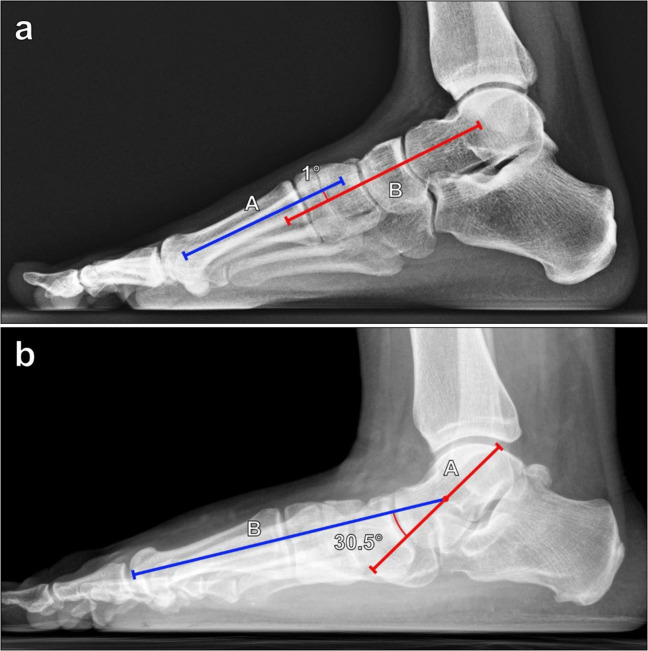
Measurement of the lateral talus-first metatarsal (Meary) angle on lateral weightbearing radiograph of the foot. a The angle is formed between lines drawn along the axis of the first metatarsal (A, blue line) and along the axis of the talus (B, red line). The long axis of the talus is either colinear or nearly colinear with the long axis of the first metatarsal, resulting in a normal angle range of 0° ± 4°. Angles greater than 4° convex downward are seen with pes planus, and angles greater than 4° convex upward are consistent with pes cavus. b In a 34-year-old male patient with posterior tibialis tendon dysfunction and flatfoot deformity (same patient as in Fig. 10b), the lateral talus-first metatarsal angle measures more than 30°, indicating borderline severe pes planus
